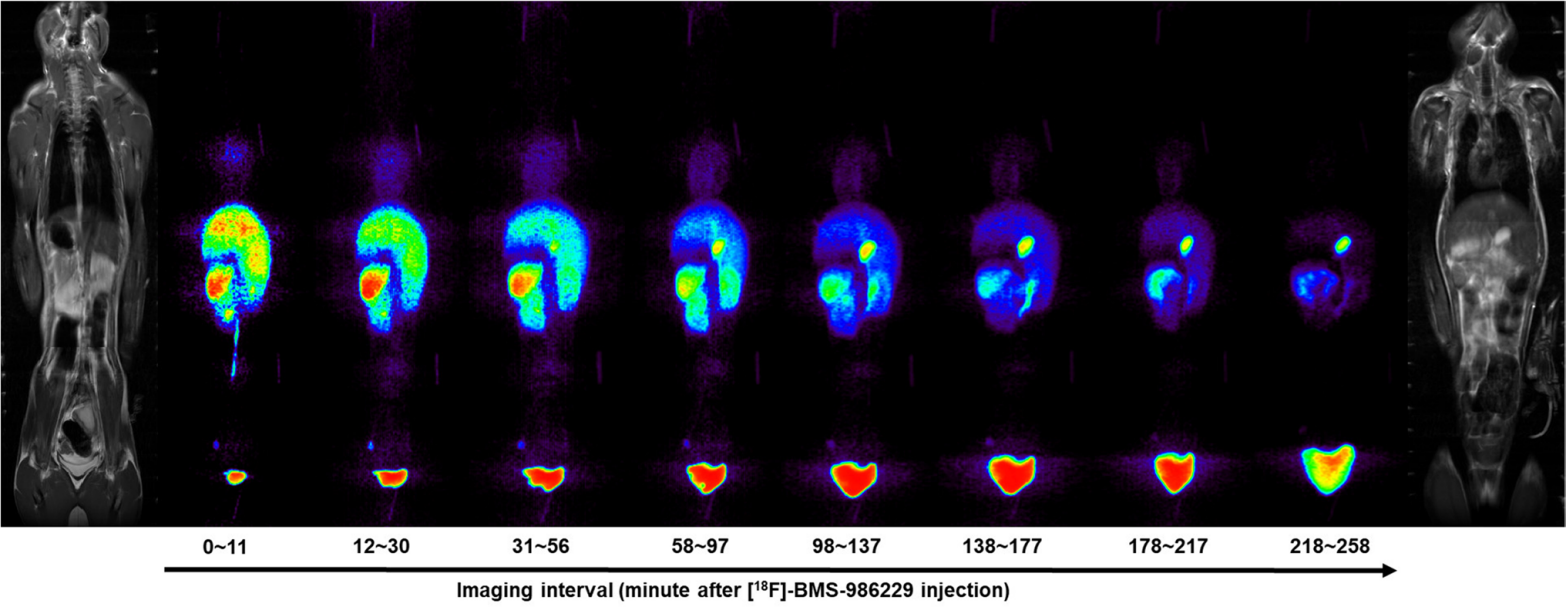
PET and MRI images in NHP. Each PET image is a non-radioactive decay corrected image
